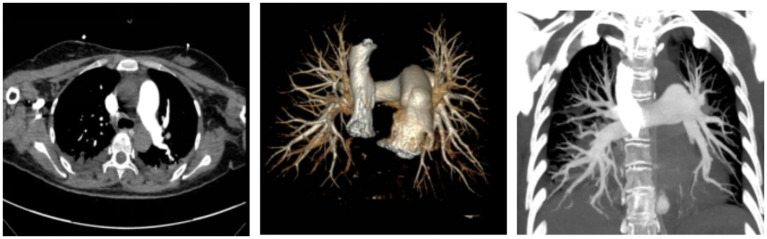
Pulmonary artery CTA findings of the patient on postpartum day 3. Demonstrating punctate filling defects in partial branches of the left pulmonary artery.
